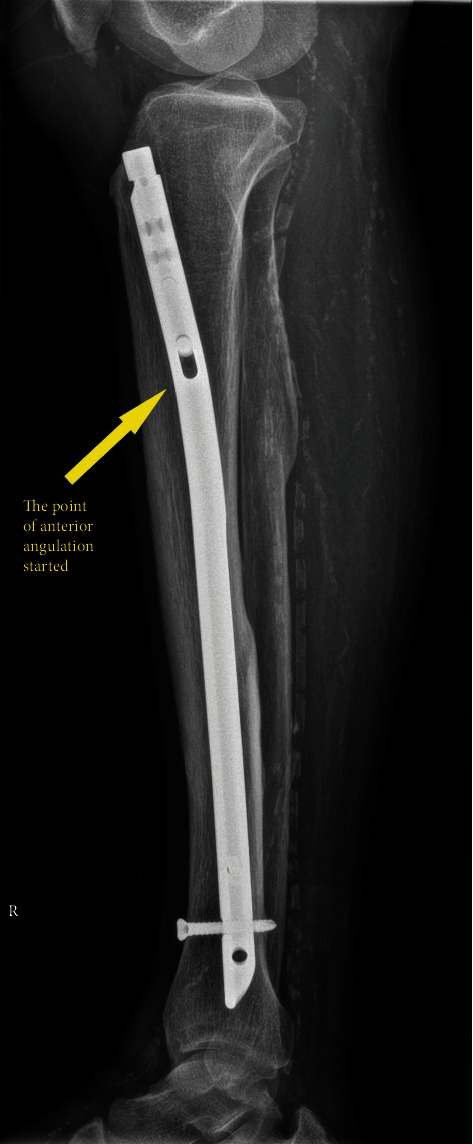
Lateral view of the tibial plain radiograph. The point of the anterior angulation started about 10 cm away from the tibial plateau.
